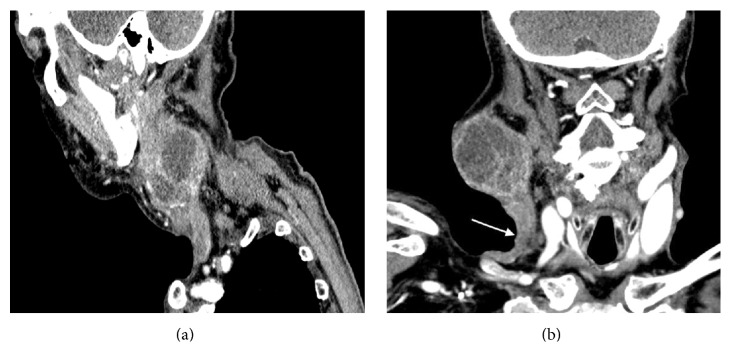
CT neck: sagittal and coronal images with contrast demonstrating parotid tumor with external jugular vein invasion with extension to the level of the right subclavian vein.
